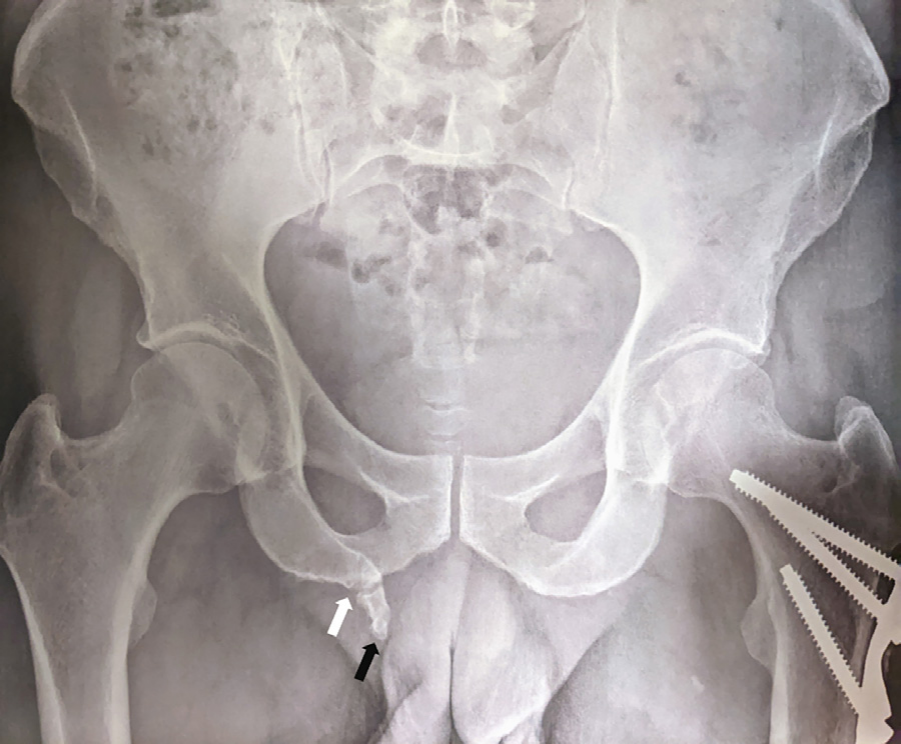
Pelvic radiography (frontal view). A mature pelvic digit, corticated (black arrow) extending from the right ischium. The proximal joint (white arrow) represents a pseudoarthrotic articulation with the ischiopubic ramus.
